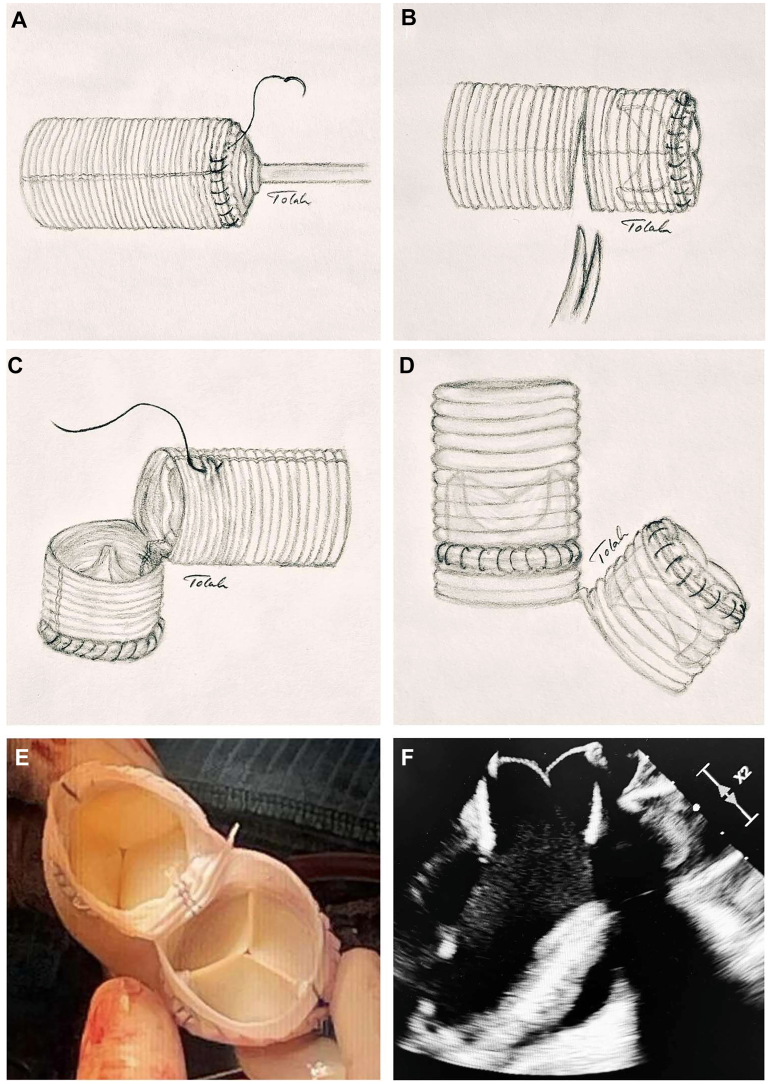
Conduit preparation: (A) Mitral prosthesis is sewn into the Dacron graft. (B) Two-thirds of the graft us trimmed above the struts. (C) The aortic prosthesis inserted and sutured 5 mm from the edge. (D) Final conduit with both prostheses. (E) Surgical and (F) echocardiographic views.
